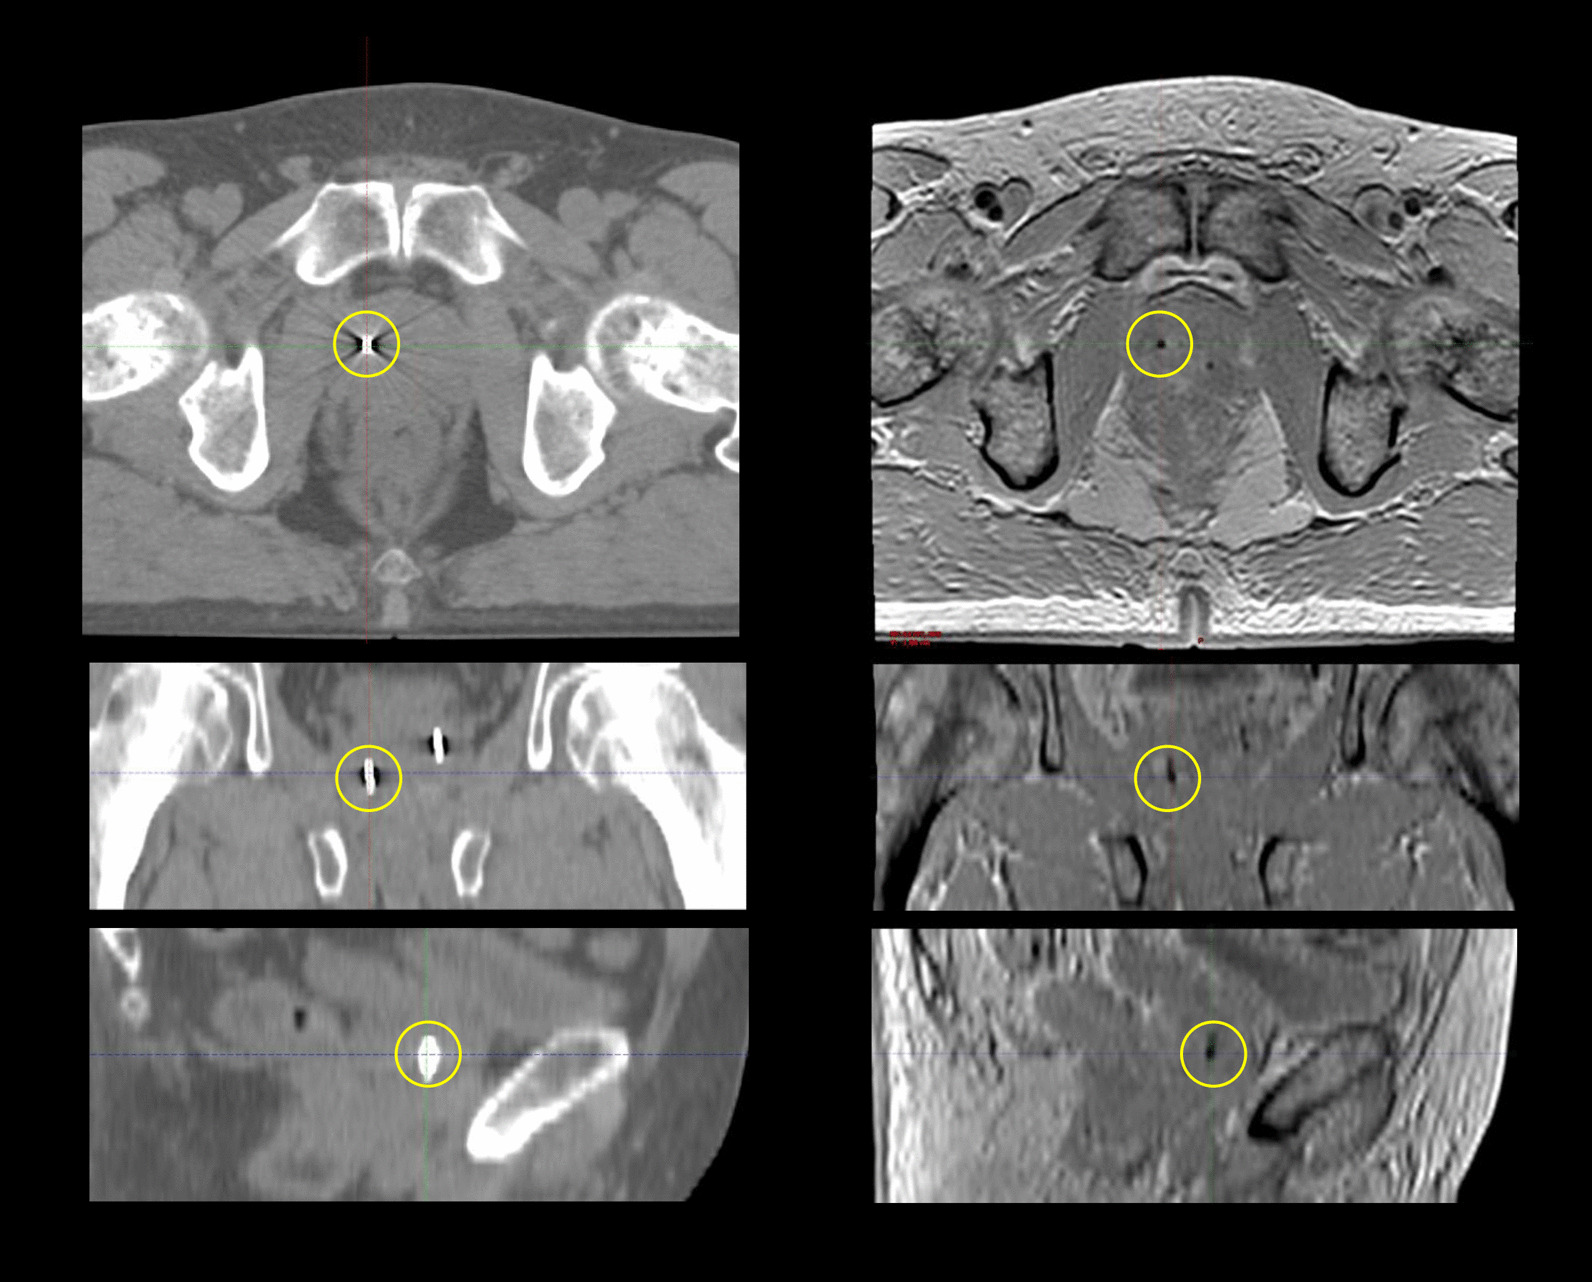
CT images (left) and multi echo gradient echo (MEGRE) MR images (right) used for gold fiducial marker (GFM) registration. The GFM, highlighted in yellow circles, generate a streak artefact in the CT images and a signal void in the MR images. Images are shown in transversal (top row), coronal (middle row, calculated projection) and sagittal (bottom row, calculated projection) planes
